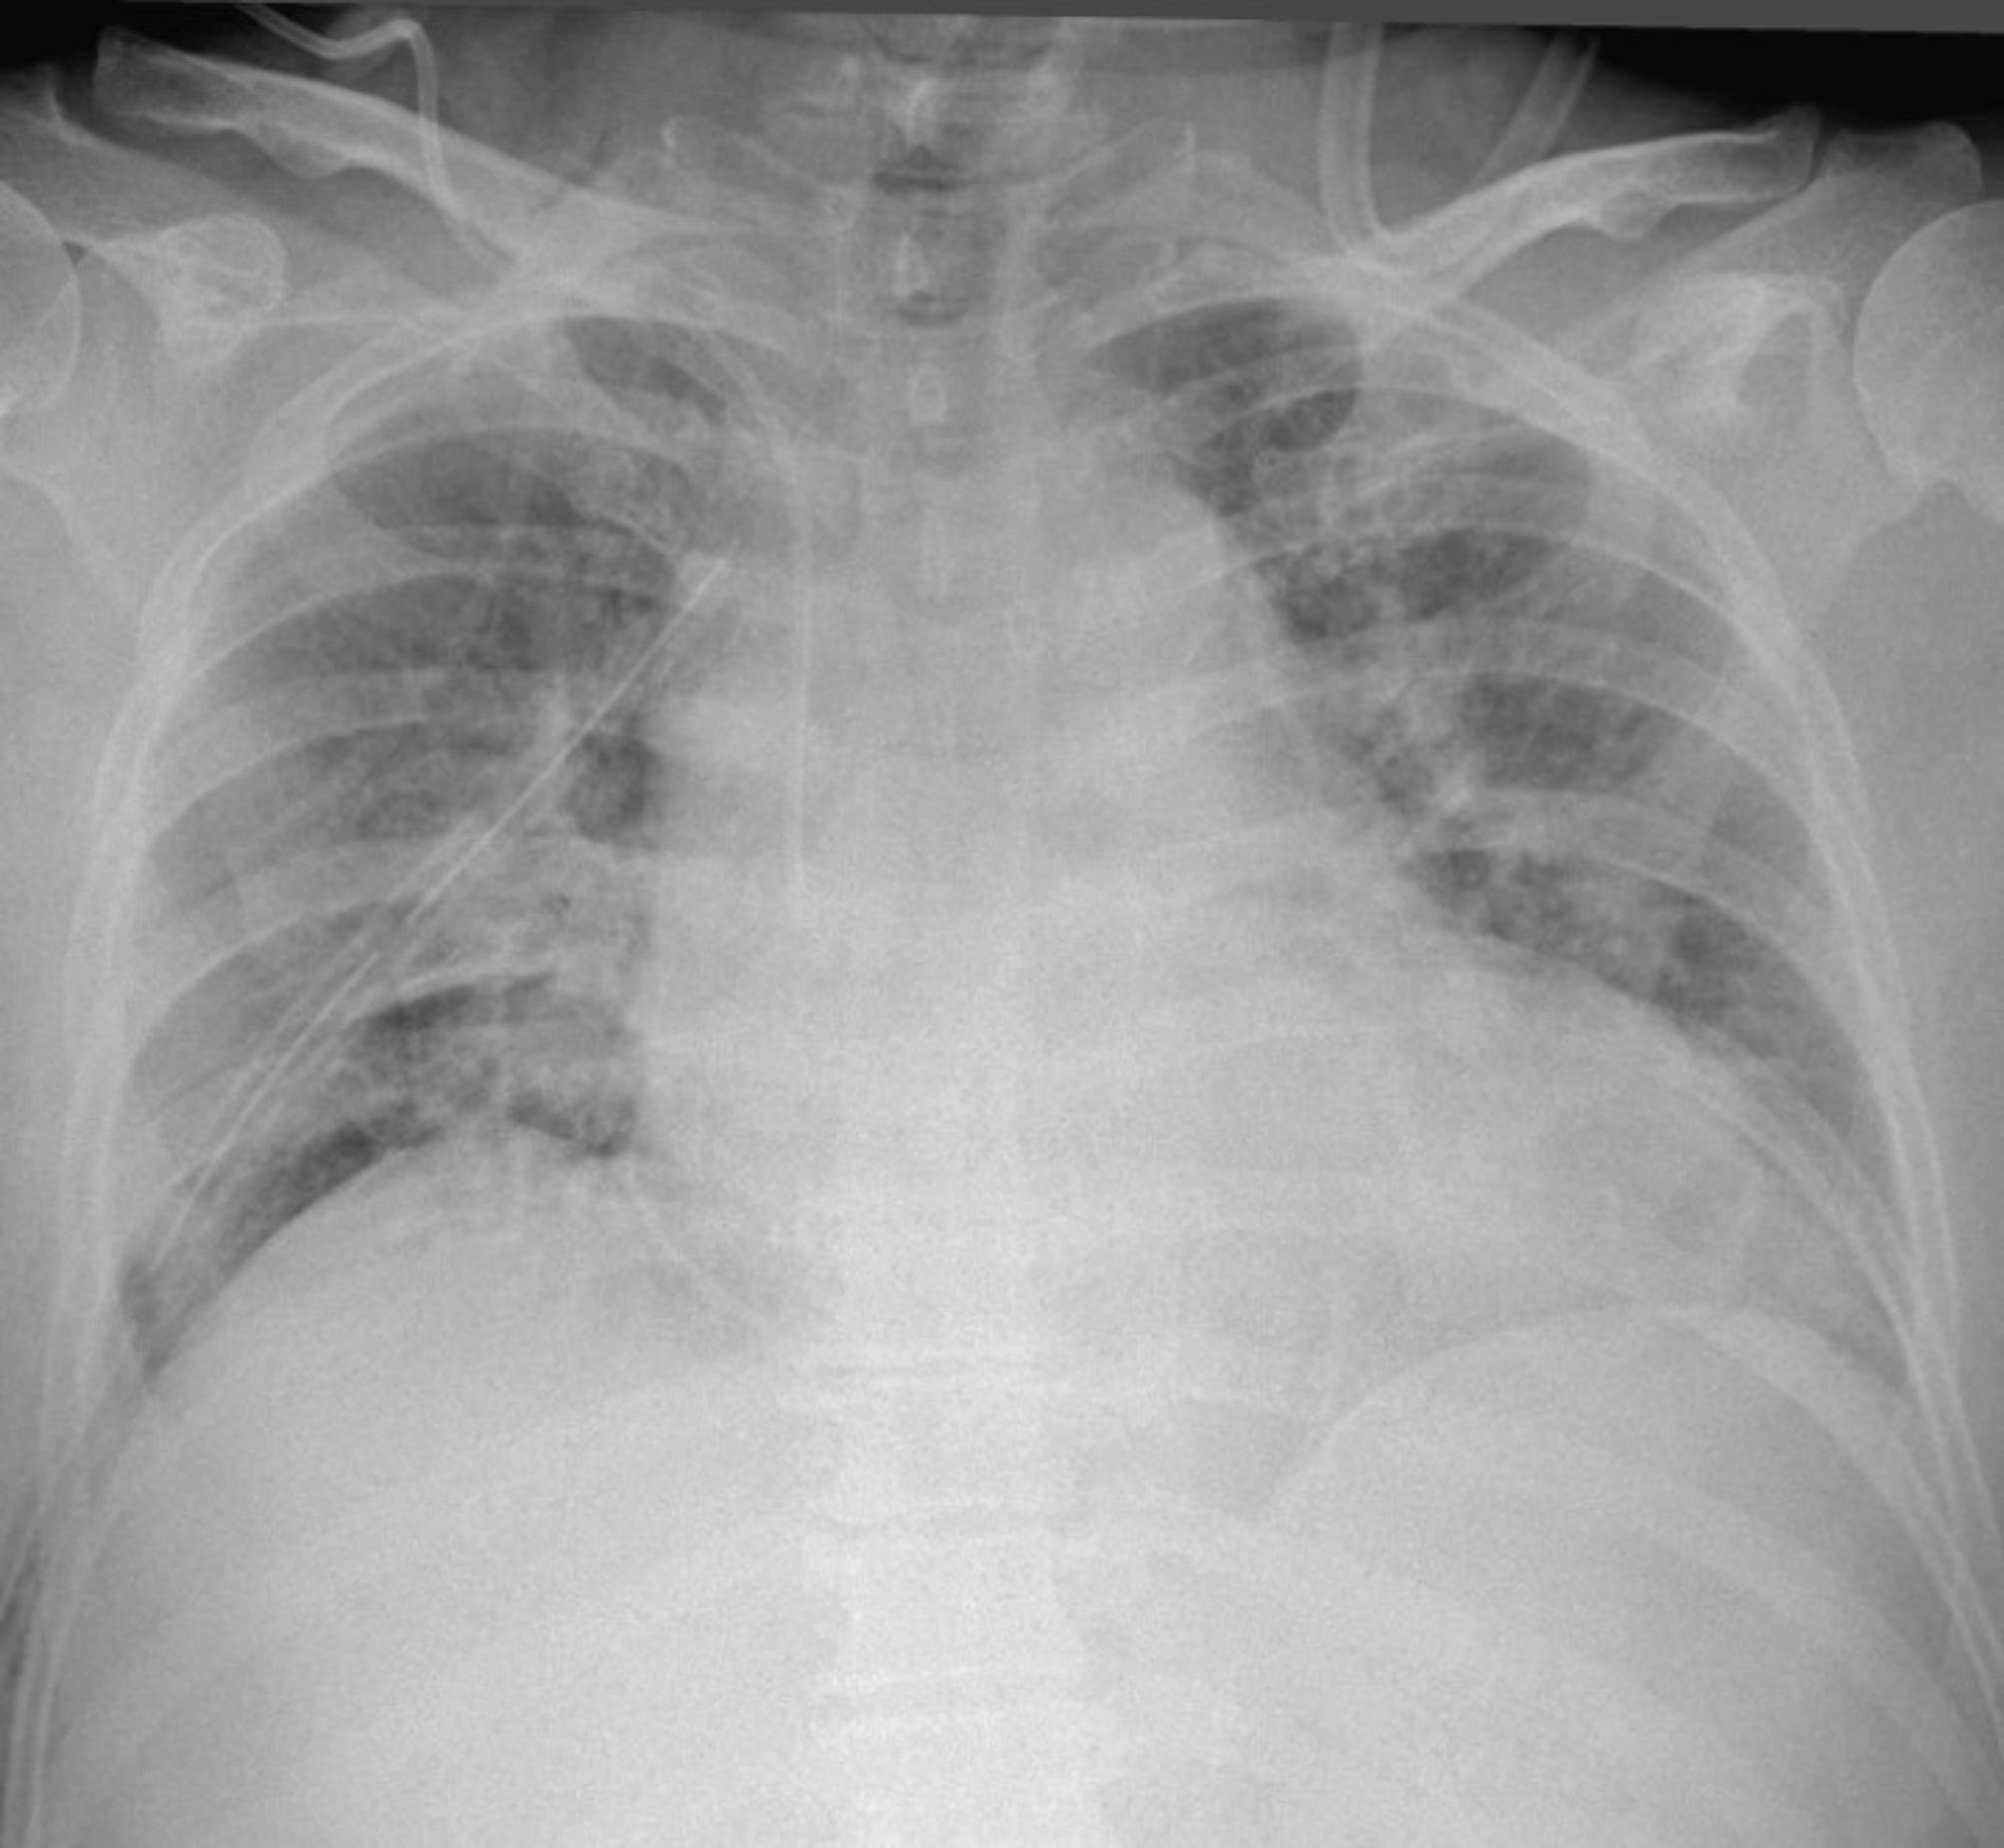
Postoperative chest X-ray (upright, anteroposterior) clear in recovery room
